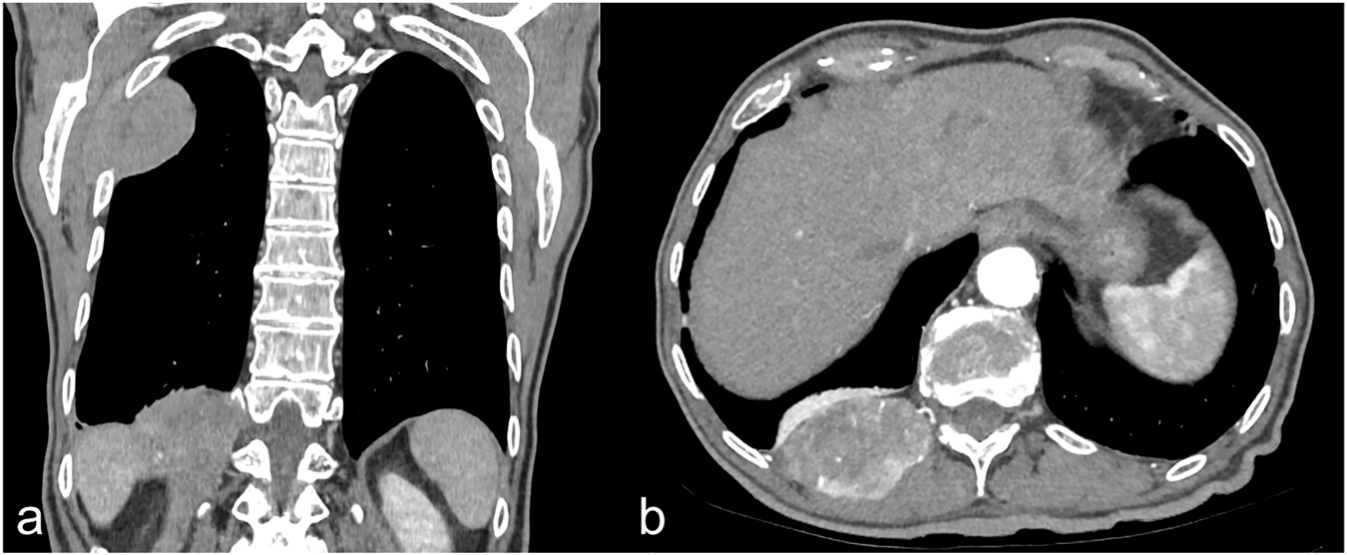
Secondary rib lesions from hepatocellular carcinoma in a patient with known liver malignancy. a Coronal CT image in the basal phase shows an oval-shaped, expansile lesion invading the rib. b Axial CT image in the arterial phase demonstrates the hypervascularization of the lesion, consistent with secondary spread of hepatocellular carcinoma
